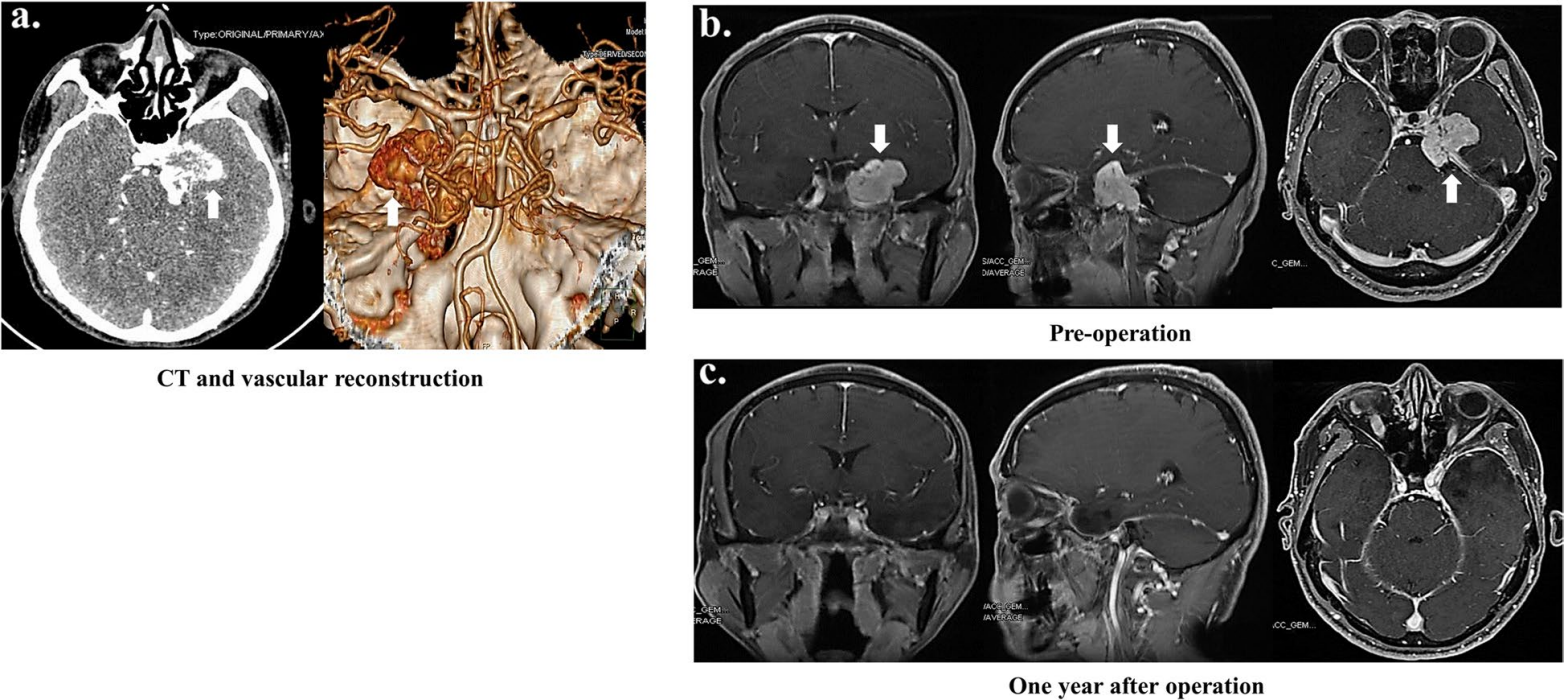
Representative CT and MRI images before and after operation. a Preoperative CT and vascular reconstruction images exhibited the SFT/HPC lesion in the left middle cranial fossa. This irregular high-density mass showing heterogeneous enhancement had unclear boundaries and destroyed the surrounding skull. And this lesion partially enveloped the left internal carotid artery segments C3-C4. b The preoperative enhanced T1WI images were displayed. The mass was located in the left middle cranial fossa, with unclear boundaries and heterogeneous enhancement. The lesion protruded locally into the left pontine corner cistern, partially compressed the left temporal lobe and brainstem and enveloped the C3-C4 segments of the left internal carotid artery. c The enhanced T1WI images of 1-year past operation were exhibited. The area of the original lesion showed postoperative changes without significant enhancement
